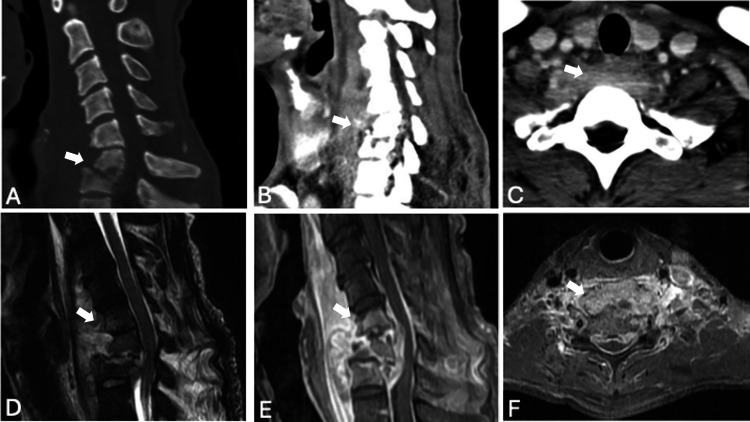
Preoperative images (A) Preoperative bone window cervical sagittal CT of the patient revealing C6-C7 bone erosion with malalignment (white arrow). (B-C) Preoperative cervical sagittal and axial CT with contrast revealing C5-C7 prevertebral abscess (white arrow). (D-F) Preoperative T2-weighted sagittal and T1-weighted sagittal and axial views with gadolinium revealing C5-C7 spondylodiscitis with epidural and prevertebral abscess (white arrow). Additionally, there is C6-C7 cord compression with signal change. CT: computed tomography
